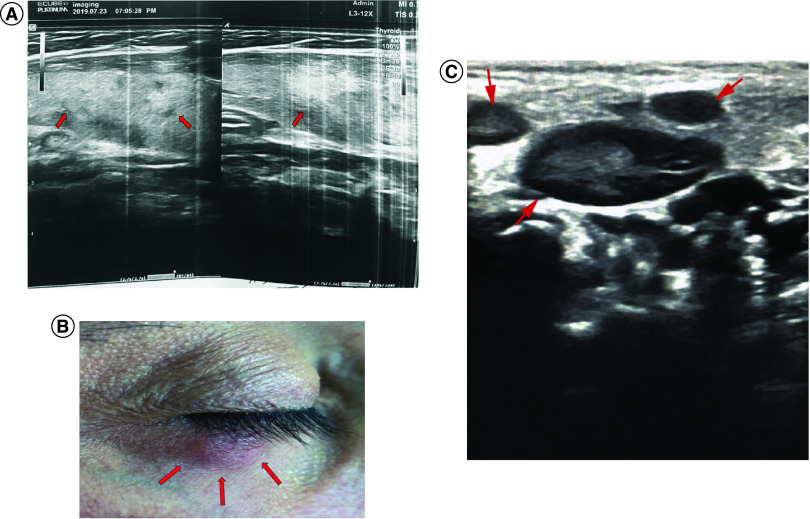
Examination of the cervical and preauricular lymphadenopathies, as well as skin lesion. (A) The ultrasound imaging in terms of the CLA detection in case 1 (maximum size = 14 mm in diameter, which has been shown with red arrays). (B) A herpes-like skin lesion represented on the right lower eyelid, as a cutaneous manifestation of COVID-19 in case 2. (C) The ultrasound imaging for inflammation-derived preauricular lymphadenopathy detection with preserved fatty hilum in case 2. The SAD regarding cortical thickness was estimated between 10–14 mm, which has been shown with red arrays. CLA: Cervical lymphadenopathy; SAD: Short axis diameter.
